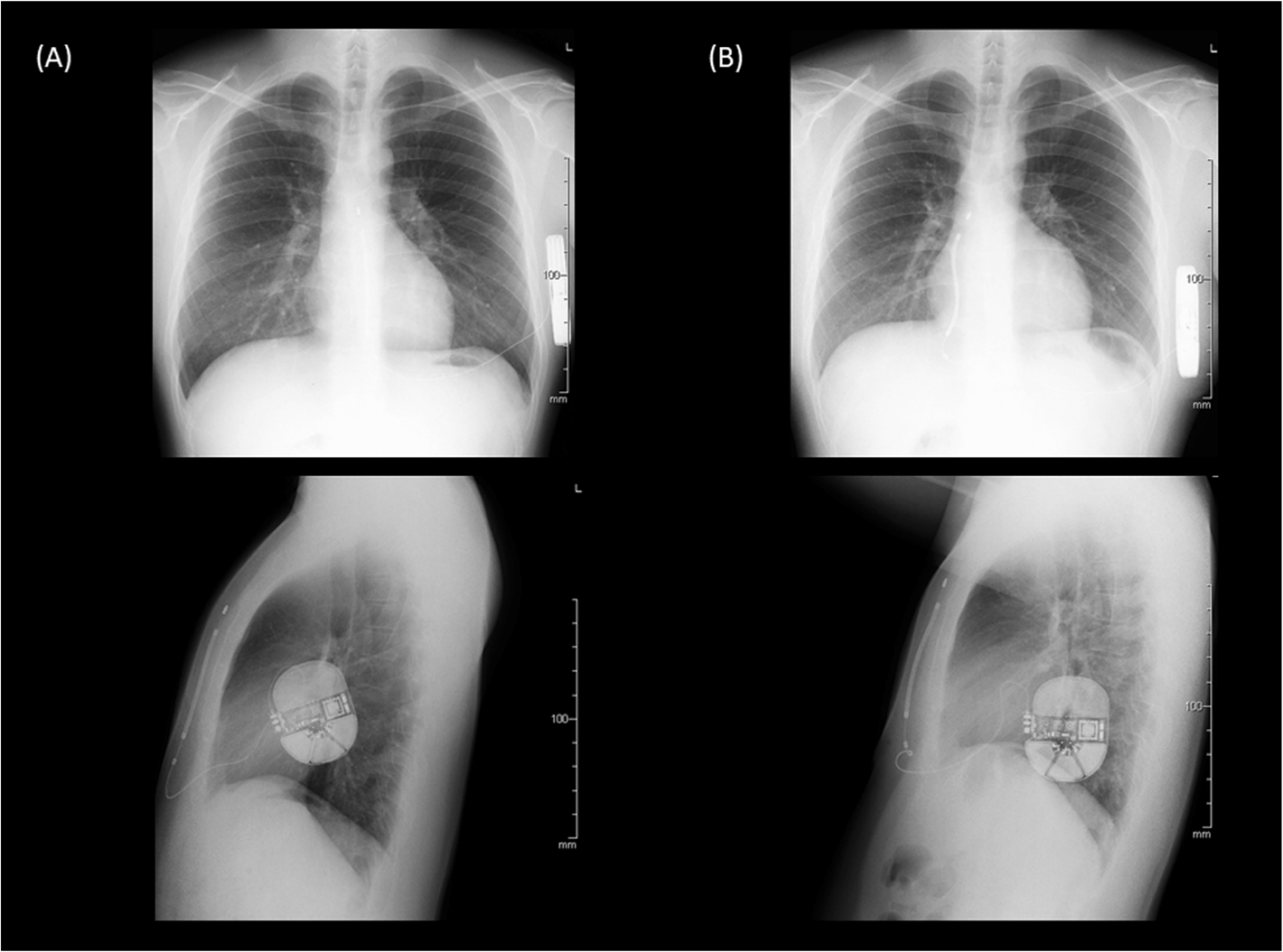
Chest X-ray (PA and RL views) before (a) and after (b) the re-implantation of the S-ICD system
